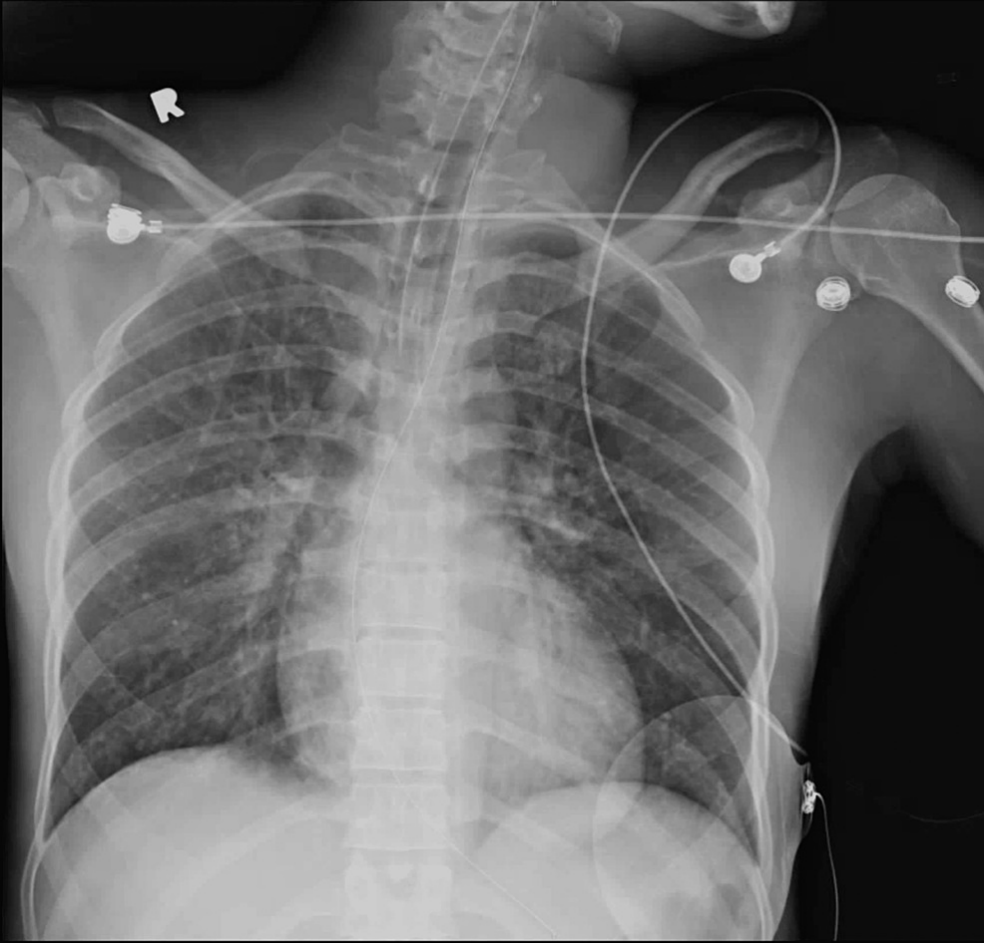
Chest X-ray after intubation (posteroanterior view)
